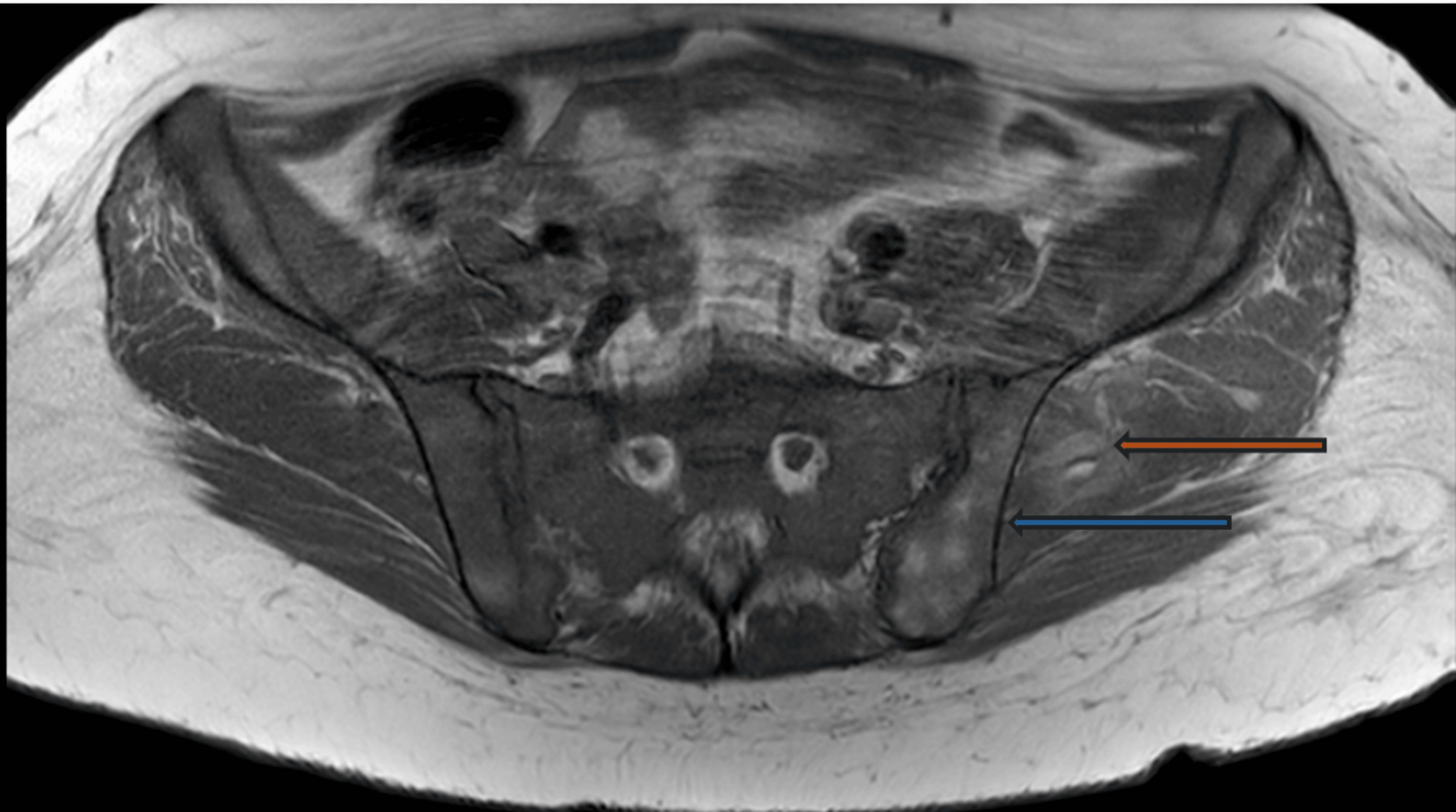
MRI abdomen and pelvis with and without IV contrast. The blue arrow showing multifocal intraosseous and orange arrow showing intramuscular hemorrhage in the left iliopsoas and gluteal regions.
